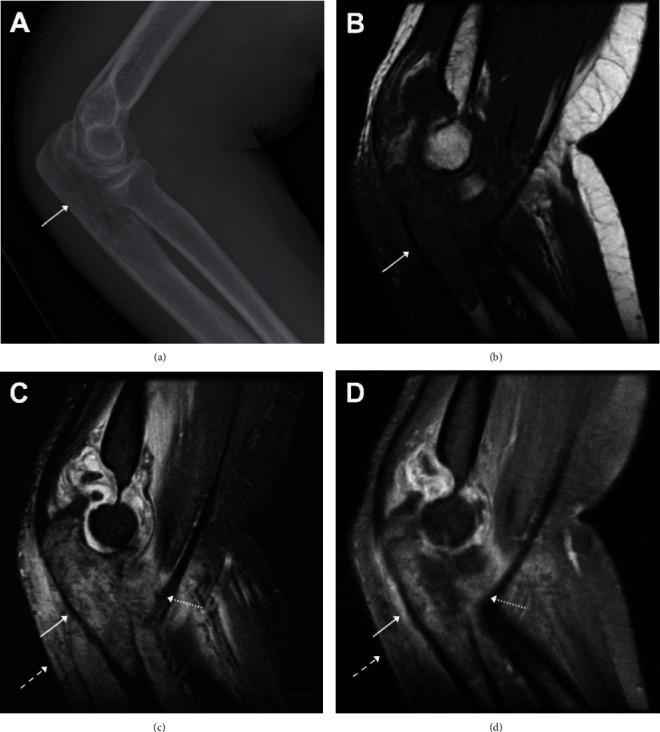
Lateral right elbow radiograph (a) shows a lytic lesion within the proximal ulna with permeative or moth-eaten appearance and wide zone of transition, suggestive of an aggressive lesion. Sagittal noncontrast T1-weighted (b), fluid-sensitive (c), and postcontrast (d) MRI images demonstrate an infiltrative mass centered within the proximal ulna (solid arrows, (b–d)) with soft tissue invasion (dotted arrow, (c, d)). There is replaced normal bone marrow signal (b), increased T2 signal (c), and postcontrast enhancement (d). The adjacent muscle demonstrates increased signal on the fluid-sensitive image (dashed arrow, (c)) with mild enhancement (dashed arrow, (d)), due to soft tissue invasion.
